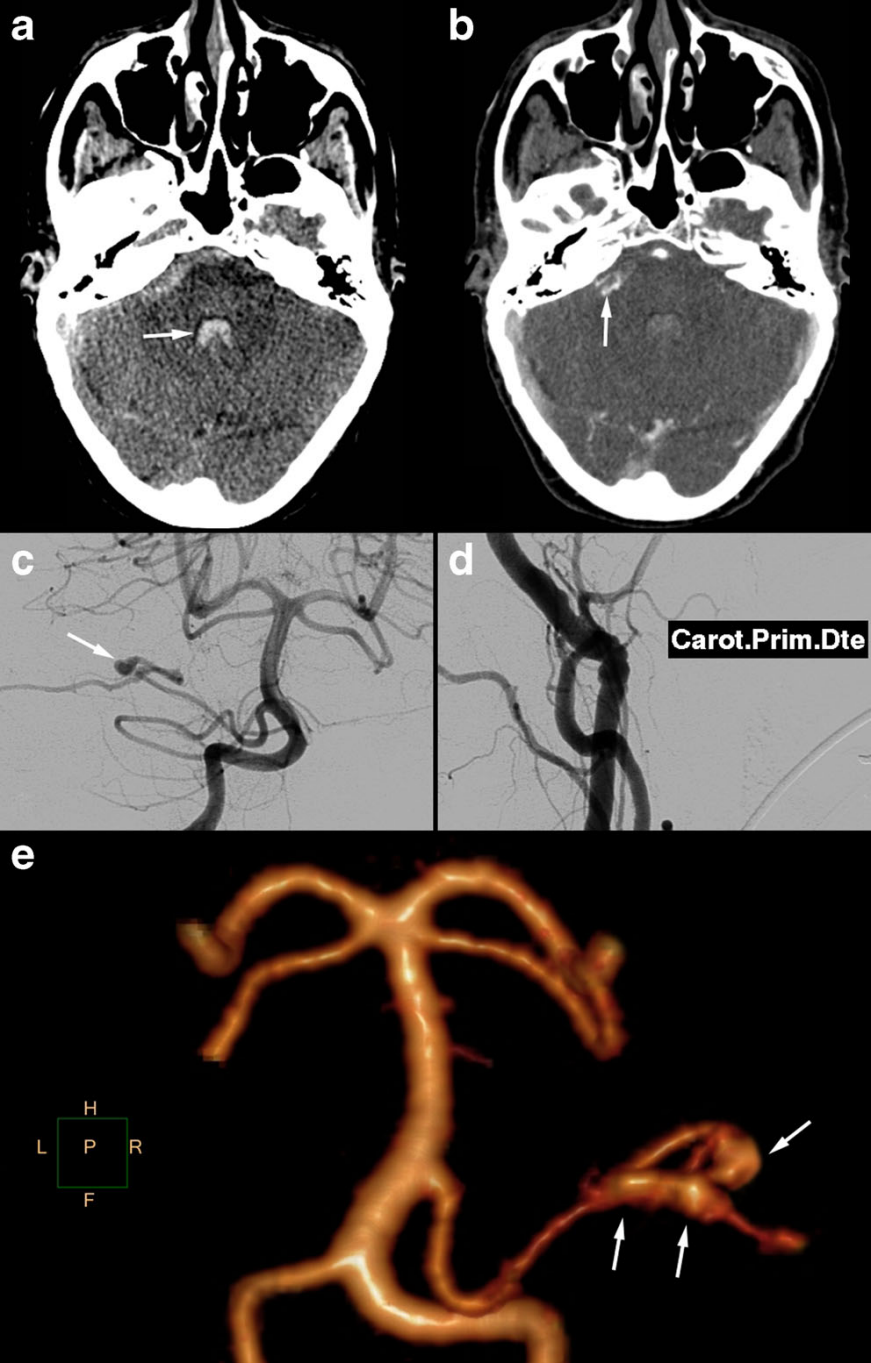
Radiologic findings in a 70-year-old female patient presenting a right-hemisphere non-traumatic SAH with tetraventricular inundation. a Cerebral non-enhanced CT showing SAH and intraventricular haemorrhage. b Cerebral CTA showing a dissecting aneurysm of the right antero-inferior cerebellar artery. c Angiogram of the right vertebral artery in arterial phase confirming the dissecting aneurysm of the right antero-inferior cerebellar artery (*white arrow*). d Right common carotid angiogram showing the typical string-of-beads aspect, in favour of FMD. e Cerebral 3D volume-rendered CTA of the dissecting aneurysm of the right antero-inferior cerebellar artery (*white arrows*)
